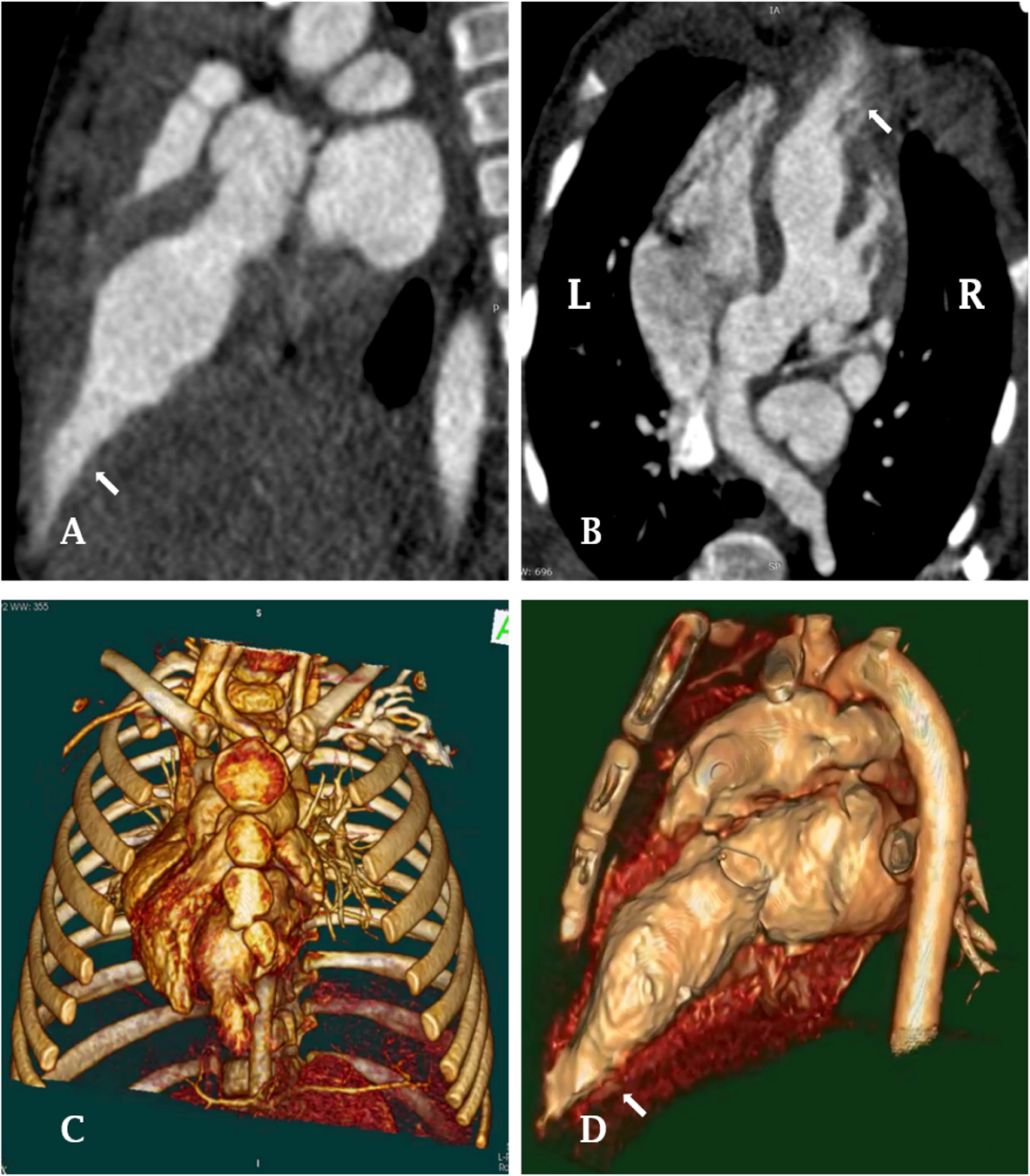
Preoperative computed tomography images of the diverticulum. Images showing a protrusion of the left ventricle (arrow) extending beyond the apex, with a wide ventricle connection (a: sagittal view; b: coronal view). A three-dimensional reconstruction showing the morphology (c) and position (d) of the diverticulum
